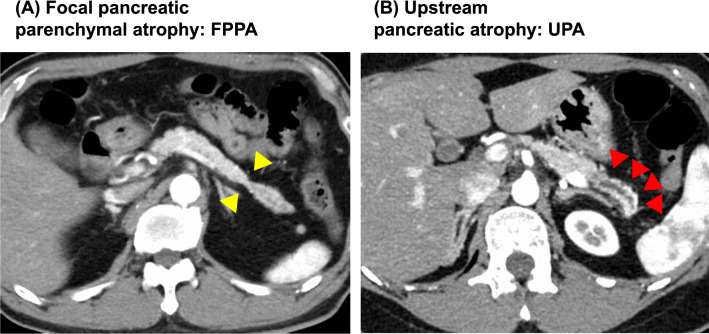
Typical CT images of atrophy of the pancreatic parenchyma in cases of carcinoma in situ. A Focal pancreatic parenchymal atrophy (FPPA) in the pancreatic tail (yellow arrows). B Upstream pancreatic atrophy (UPA) in the pancreatic tail (red arrows)
